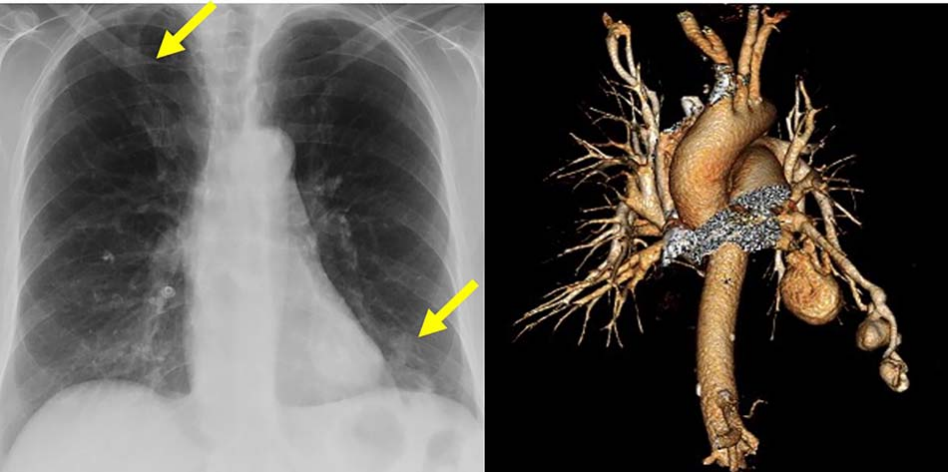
Chest radiograph and computed tomography volume rendered images of multiple aneurysmal PAVMs, including three in lingula and one in right upper lobe. Yellow arrows denote aneurysms.
